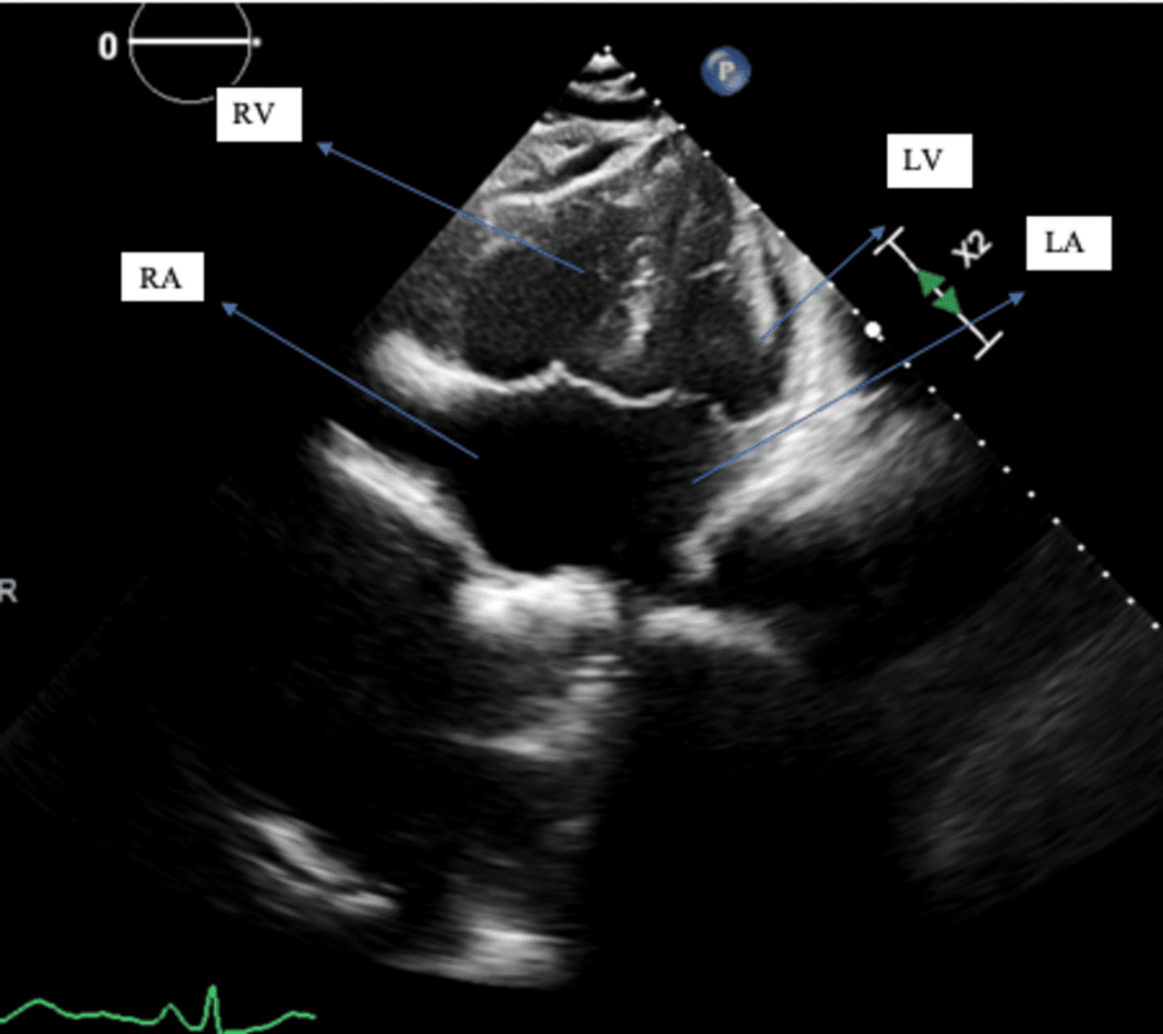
Transthoracic echocardiogram (apical four-chamber view) showing McConnell’s sign, which is akinesia of the right ventricular lateral wall with sparing of the apex. LA, left atrium; LV, left ventricle; RA, right atrium; RV, right ventricle
